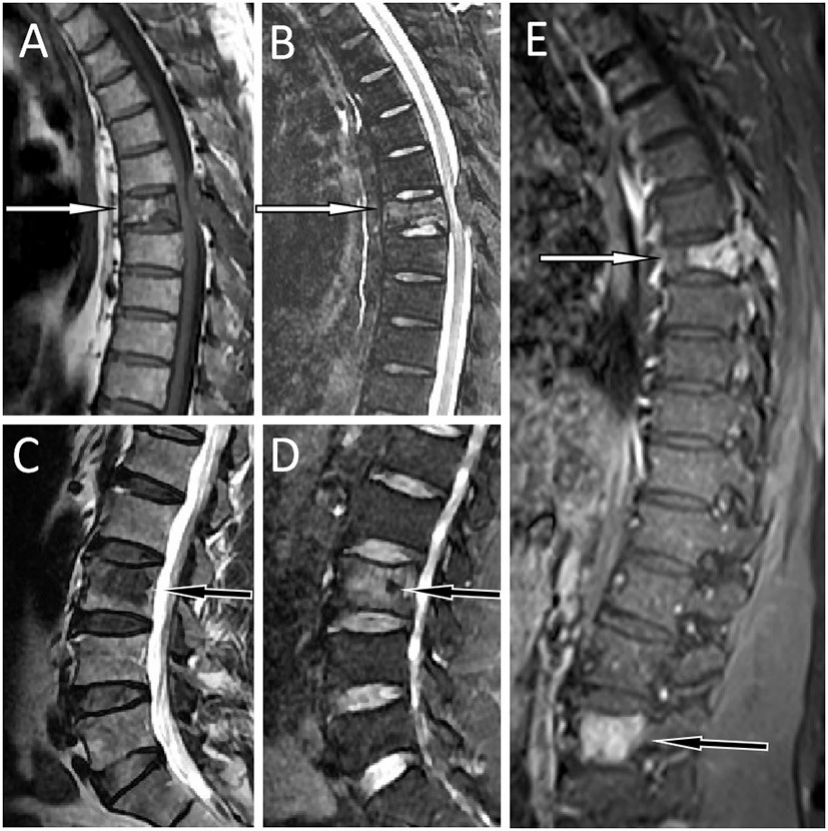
MRI showed abnormal signal shadows in the 7th thoracic (T7) and adnexa vertebrae [(A) T1WI; (B) T2WI fat suppression sequence; white arrows] and the 3rd lumbar vertebrae [(C) T1WI; (D) T2WI fat suppression sequence; black arrows]. Besides, The T7 vertebrae became wedge-shaped, suggesting a pathological compression fracture. T1WI contrast enhancement showed obvious homogeneous enhancement of the lesions (E). T7, the white arrow; L3, the black arrow.
